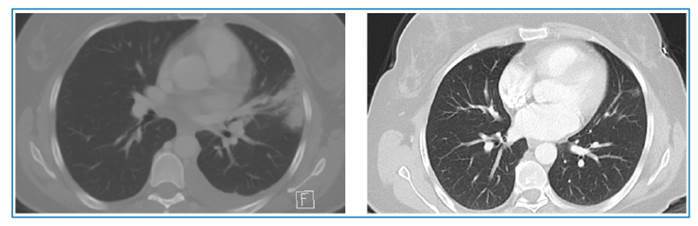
Thoraciccomputed tomography images taken one month later showing complete regression of the lesions in the lung parenchyma.
